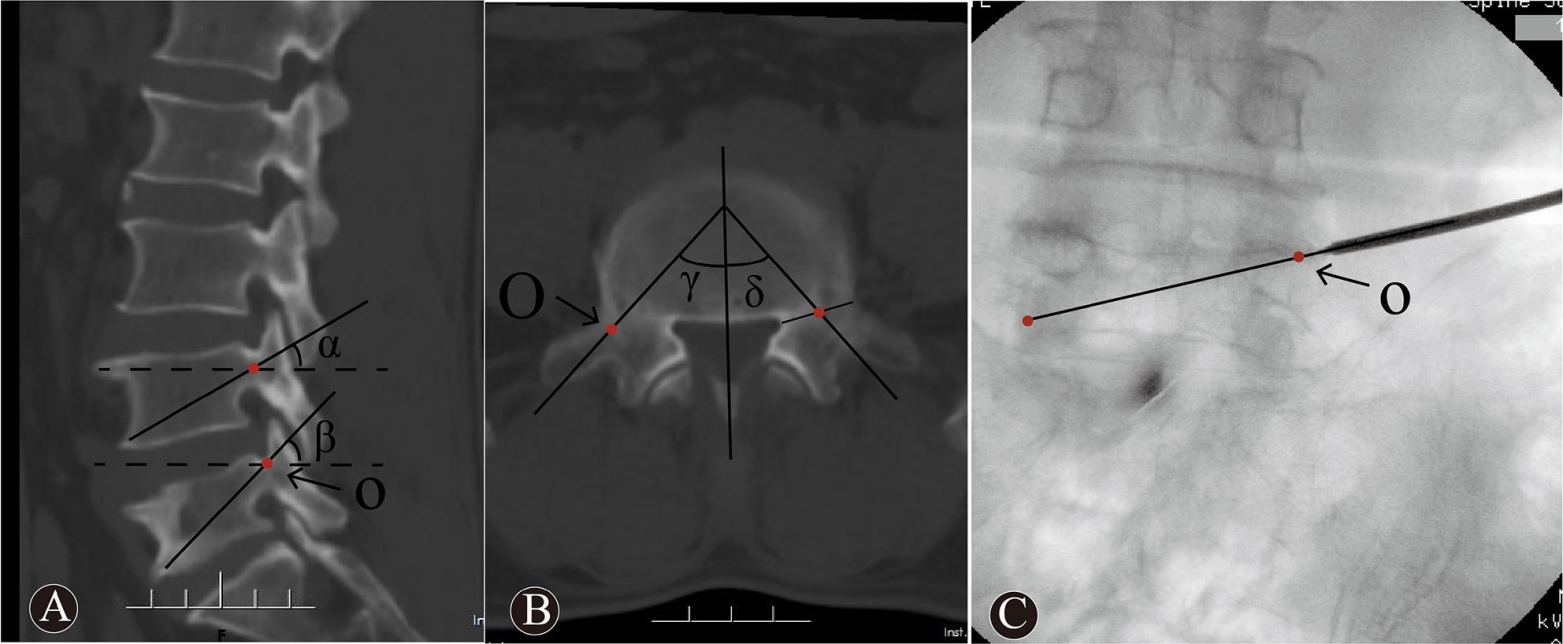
Images showed the puncture approach via point “O” and TPA. The sagittal CT image (A) was showed that α and β were sagittal puncture angle of T-PKP and O-PKP, respectively. The cross-sectional CT image (B) was showed that γ and δ were transverse puncture angle of O-PKP and T-PKP, respectively. The X-ray image (C) was showed the puncture approach of O-PKP.
